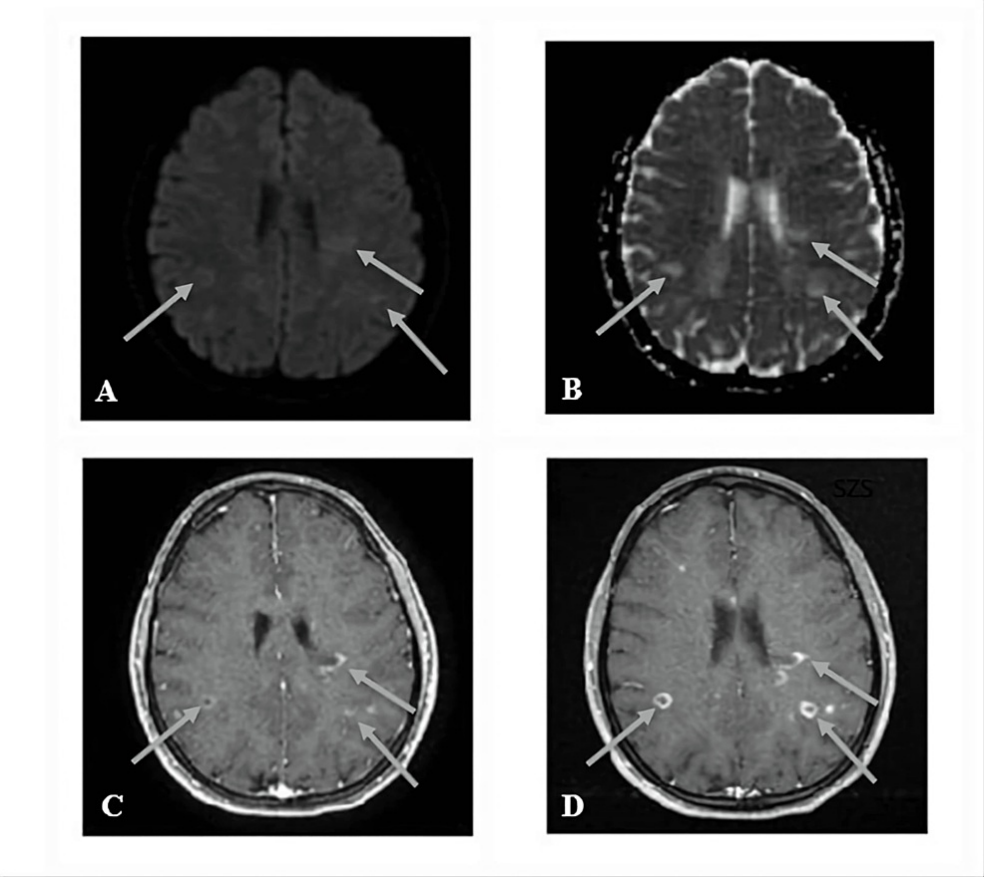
A 23-year-old female MS patient with multiple supratentorial brain parenchymal lesions (grey arrows). (A and B) Axial DWI and ADC map showed hyperintensity of the lesions. (C) Axial MPRAGE` with early enhancement showed faint enhancement. (D) Delayed enhancement of the lesions showing intense enhancement activity. MS, multiple sclerosis; DWI, diffusion-weighted image; ADC, apparent diffusion coefficient; MPRAGE, magnetization-prepared rapid acquisition gradient echo
